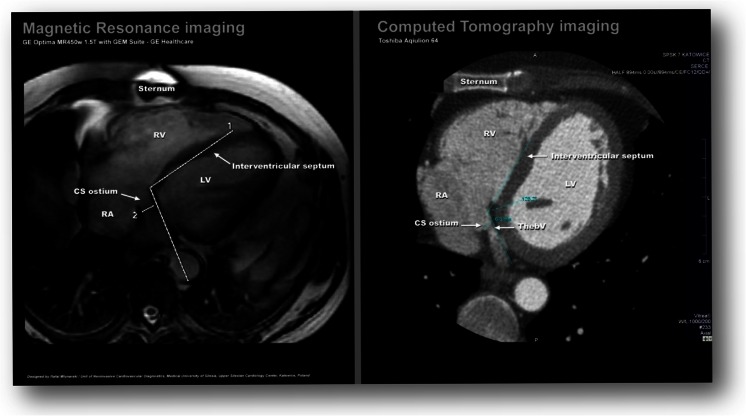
Graphic comparison of the visualization of the coronary sinus ostium in computed tomography as well as CMR. *RA* right atrium, *LV* left ventricle, *CS* coronary sinus
